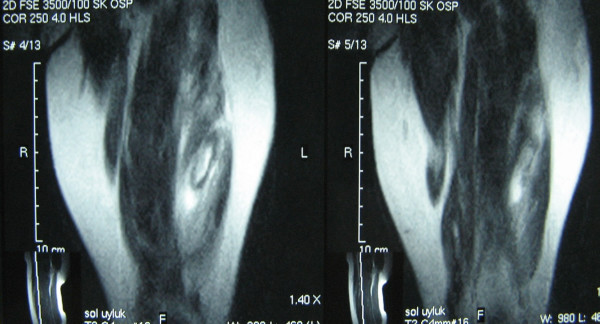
Coronal MR images show hemorrhagic and rupture sites.
